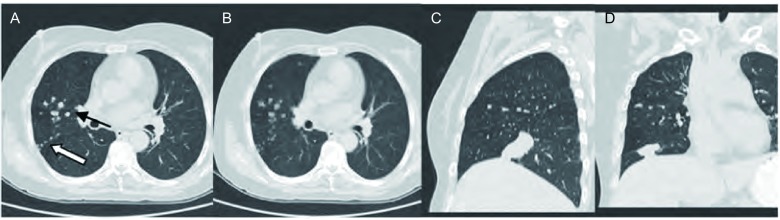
患者女，60岁，右肺下叶腺癌伴干性胸膜转移（叶间胸膜小结节型）。A、B：右侧叶间胸膜多发小结节，结节部分沿右肺水平裂呈簇状分布，部分沿右肺斜裂排列呈串珠状排列（层厚分别为5 mm和2 mm）。C、D（同一患者MPR）：原发灶位于右肺下叶，并与右肺斜裂关系相贴；右侧叶间胸膜小结节，沿右肺水平裂呈串珠状排列（层厚分别为5 mm和2 mm）。 Female, 60-year-old computed tomography (CT) images show dry pleural dissemination (DPD) (the small interlobar pleura nodules) in 60-year-old woman with lung adenocacinoma. A, B: Lung window of CT image (5-mm, 2-mm section thickness) shows multiple small interlobar pleura nodules within right minor and major fissures which arranged along the interlobar pleura as beaded string or in clusters around the interlobar pleura. C, D: The primary tumor in the right lower lobe is visualized, and located adjacent to the right major fissure. CT images (5-mm, 2-mm section thickness) show multiple small interlobar pleura nodules which arranged along the right minor fissure as beaded string.
